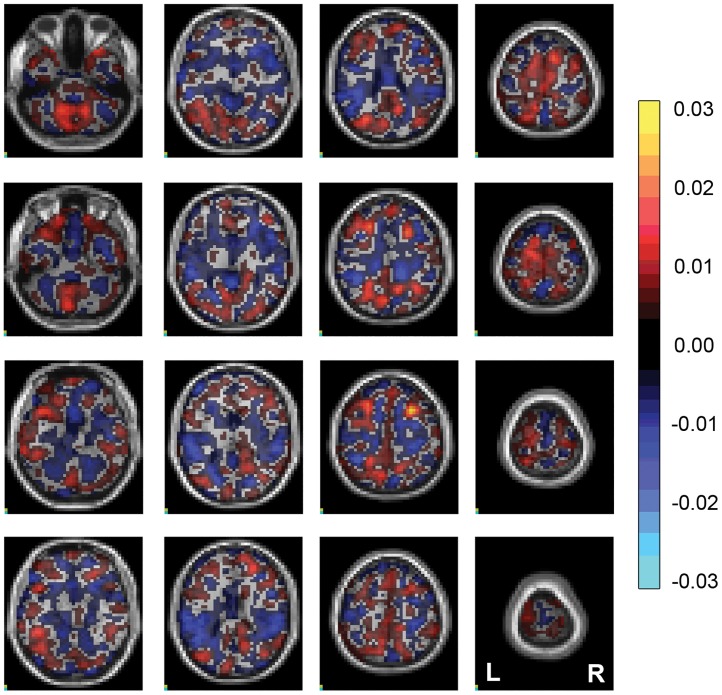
Weight maps for the prediction of the principal component 1. The slices contain the weight maps overlaid onto a standard MRI volume. Areas in red represent positive weights (metabolism correlates directly with the component) and areas in blue represent negative weights (metabolism correlates inversely with the component). L = left, R = right.
